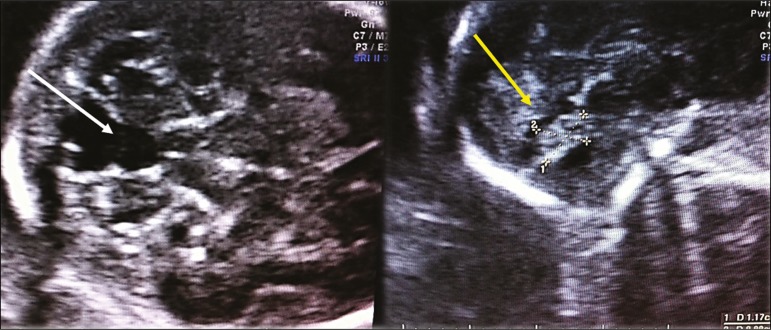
Axial ultrasound of a fetus with vermian hypoplasia, showing communication between the fourth ventricle and the cisterna magna (white arrow). Sagittal ultrasound showing a small vermis, an abnormal fastigium, and the tentorium in a normal position (yellow arrow).
